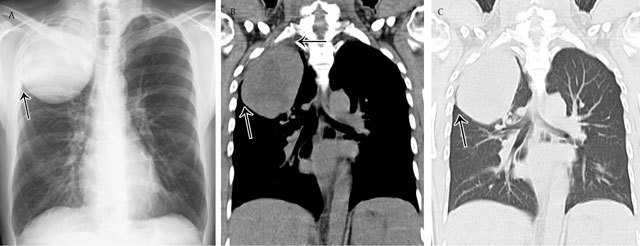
Parenchymal opacity. Adenocarcinoma of the right upper lobe – Frontal chest radiograph (a) shows a large mass in the right upper lobe presenting acute angles (black arrow) with the pleura and chest wall. Corresponding coronal reformatted CT image in mediastinal (b) and lung window (c).
